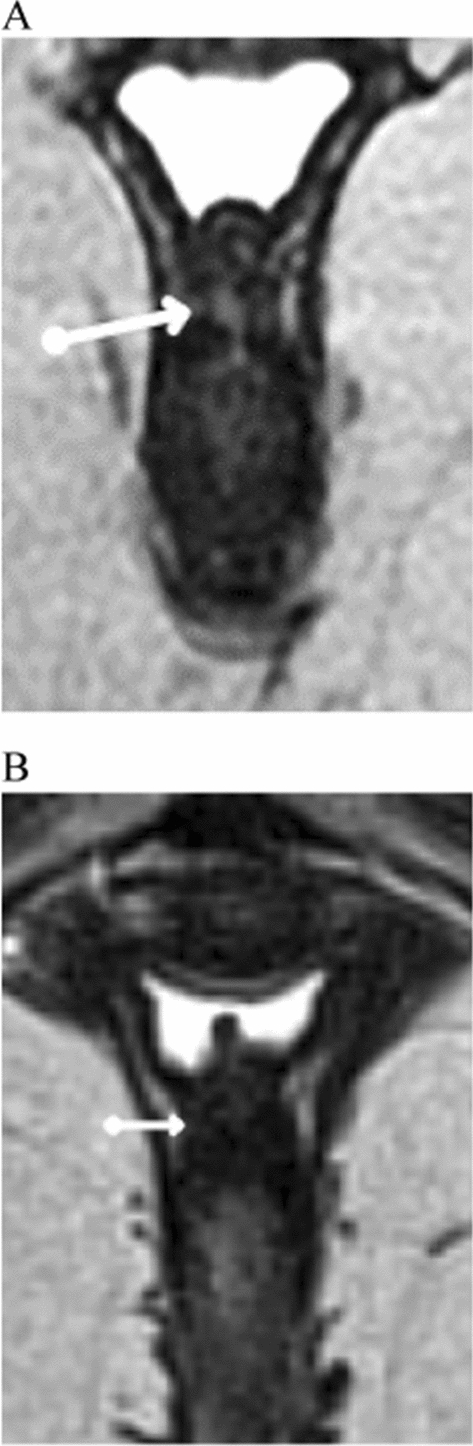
(Patient11)—Axial T2-weighted magnetic resonance image shows a fistulous track (white arrow) between the anal canal and the posterior vaginal wall (A). Magnetic resonance image after laser therapy no longer identifies the fistulous track with presence of low signal intensity tissue on T2-weighted images (white arrow), inferring fibrosis (B)
